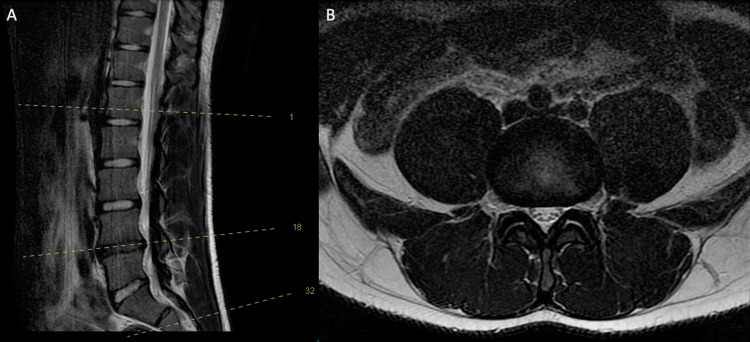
Magnetic resonance imaging (MRI) of the lumbar spine A: Sagittal view with reference line; B: Axial view at the L4-L5 level
